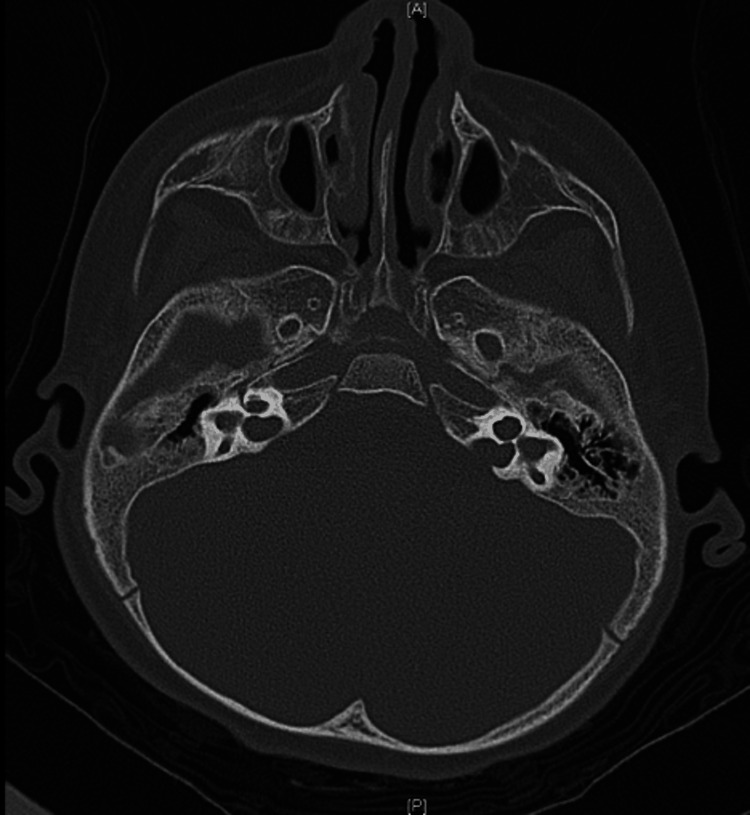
CT temporal bone axial cut showing patent bilateral internal auditory canals with dilated cystic vestibules, and dilated cochlear middle and apical cochlear turns.
